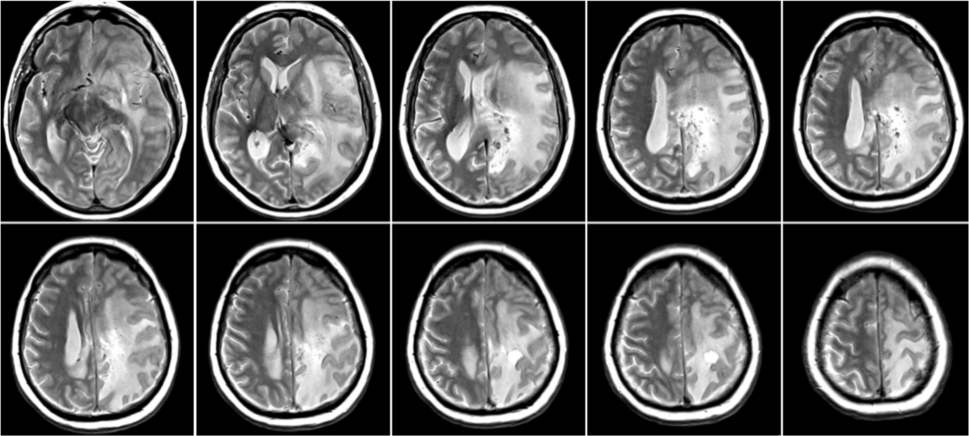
T2-weighted MRI 6 weeks after the initial MRI and after tapering off glucocorticoids. Note the more pronounced midline shift to the right as compared to the prior MRI
